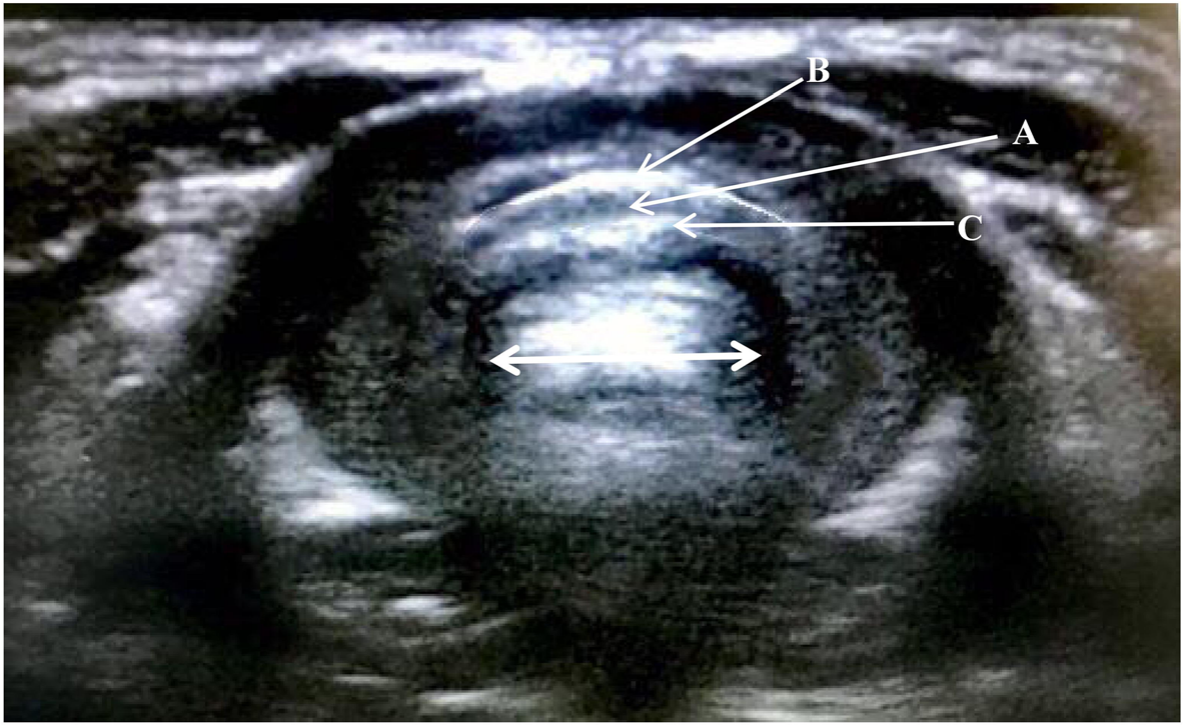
The anatomy of the airway at the level of the cricoid cartilage in the ultrasound image. The cricoid cartilage is a round hypoechoic structure (A) with hypoechoic edges (B and C).The air-column appeared hypoechoic and created a posterior acoustic shadow. The mucosa-air interface created a hypoechoic edge. Solid arrow represents the transverse diameter of the air-column at the level of cricoid cartilage
